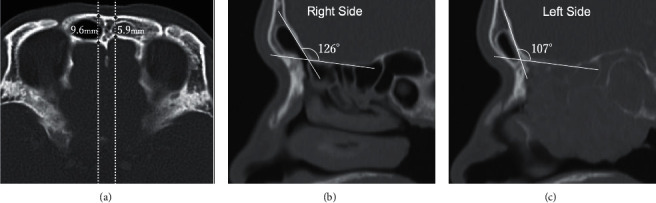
Computed tomography of the frontal sinus. (a) Axial section. The anteroposterior (AP) diameter of the right frontal sinus is 9.6 mm and that of the left frontal sinus is 5.9 mm. (b, c) Sagittal section of the right and left frontal sinuses. The skull base-frontal sinus angle (SBA) on the right frontal sinus is 126° and that of the left frontal sinus is 107°.
